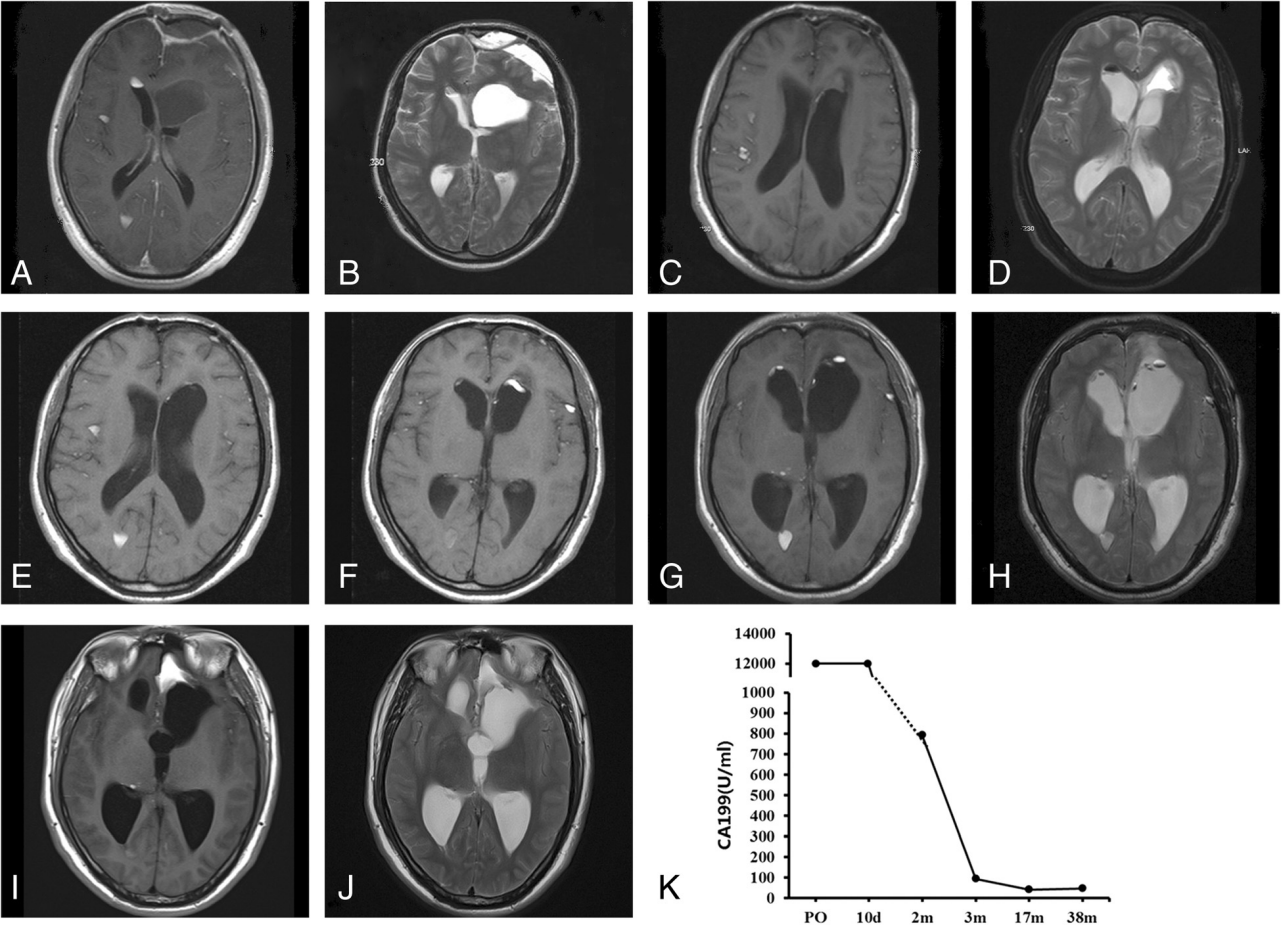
Follow-up of the patient. MRI scans half a month post-surgery (a, b), one month post-surgery (c, d), half a year post-surgery (e, f) and one and half a year post-surgery (g, h) showed that the size of residual tumor decreased gradually, which correlated with the trend of decreased value of CA 199 (k). While the latest MRI scan three years post-surgery (i, j) showed an enlargment of the lesion in the left anterior cranial base, consistently, the value of CA 199 raised up from 41.7U/ml to 47.0 U/ml. PO, pre-operation
